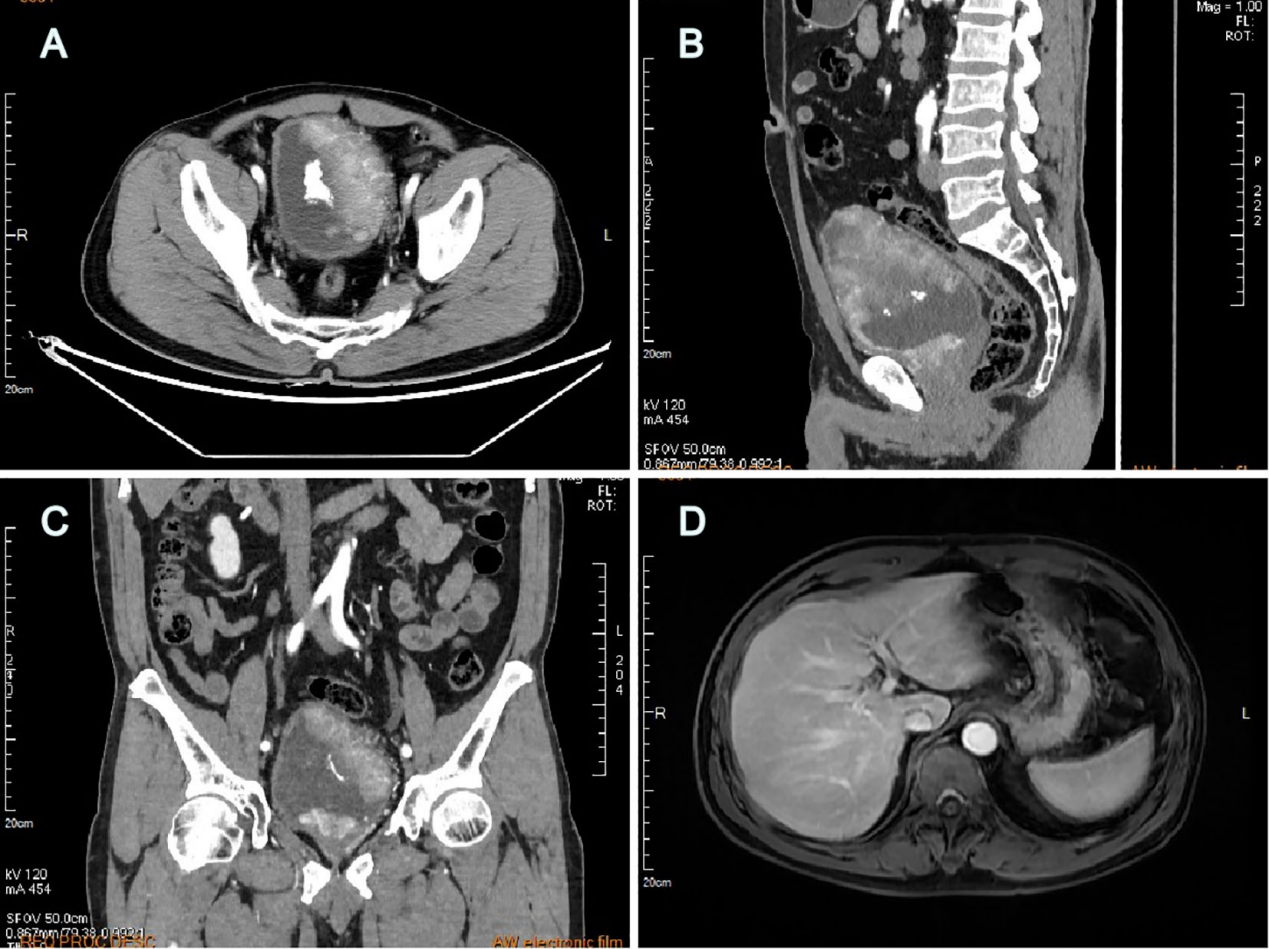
Computed tomography urography revealed multiple large lesions of the bladder (A) transverse plane, (B) sagittal plane, (C) coronal plane; Abdominal MRI with contrast revealed no detectable liver lesions (D).
